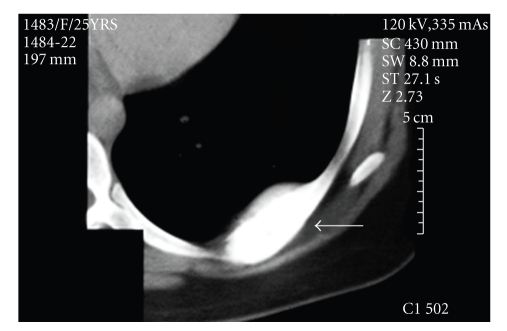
Computed tomography demonstrates a mass in the rib.
